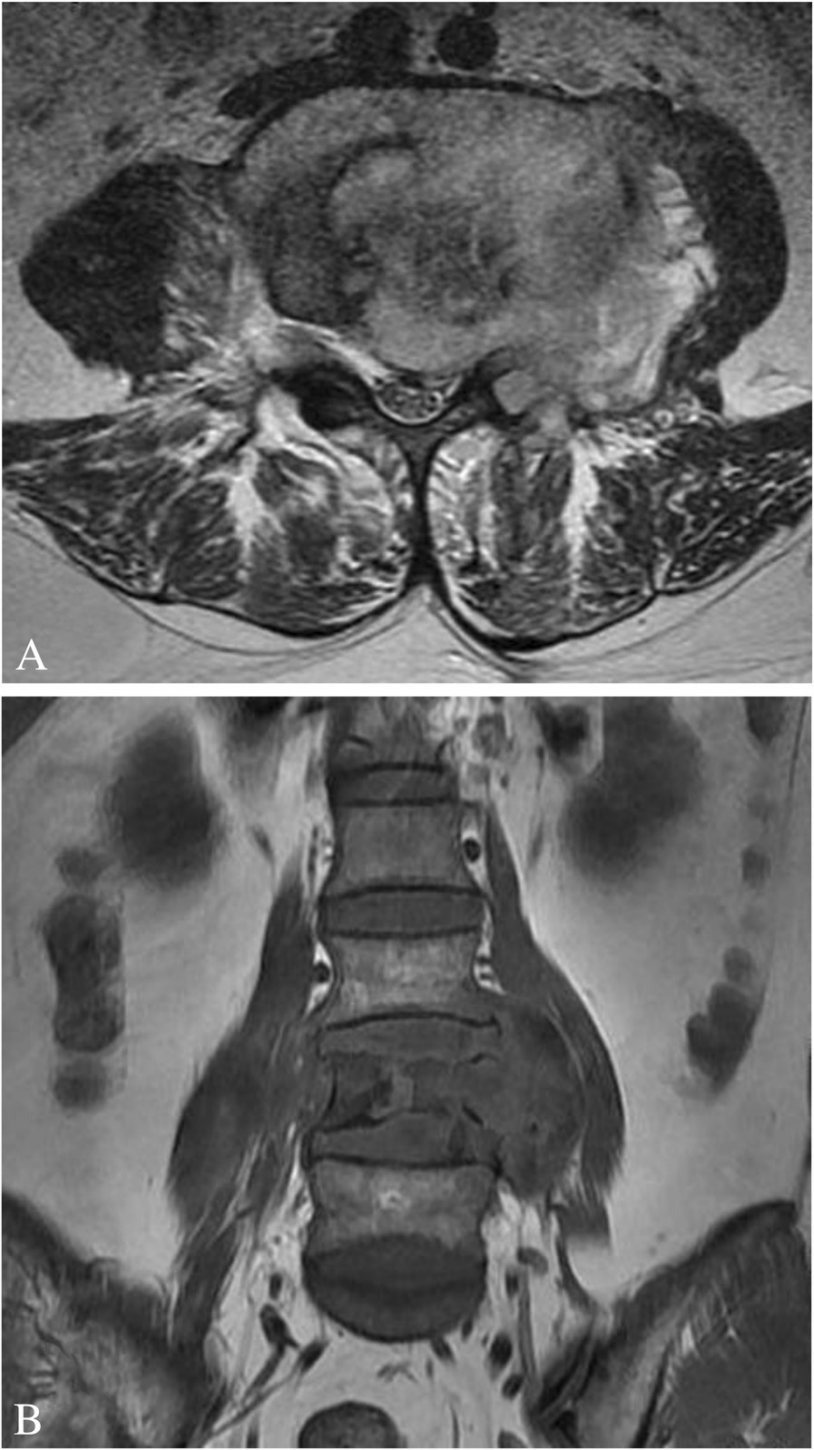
MRI of the lumbar vertebrae. (A) Transverse section imaging of L4 showed the involvement of left pedicle and transverse process, the paravertebral soft tissue mass and the spinal canal stenosis. (B) Coronal section imaging of lumbar vertebrae showed the osteolytic lesions of vertebral body at L4 level.
